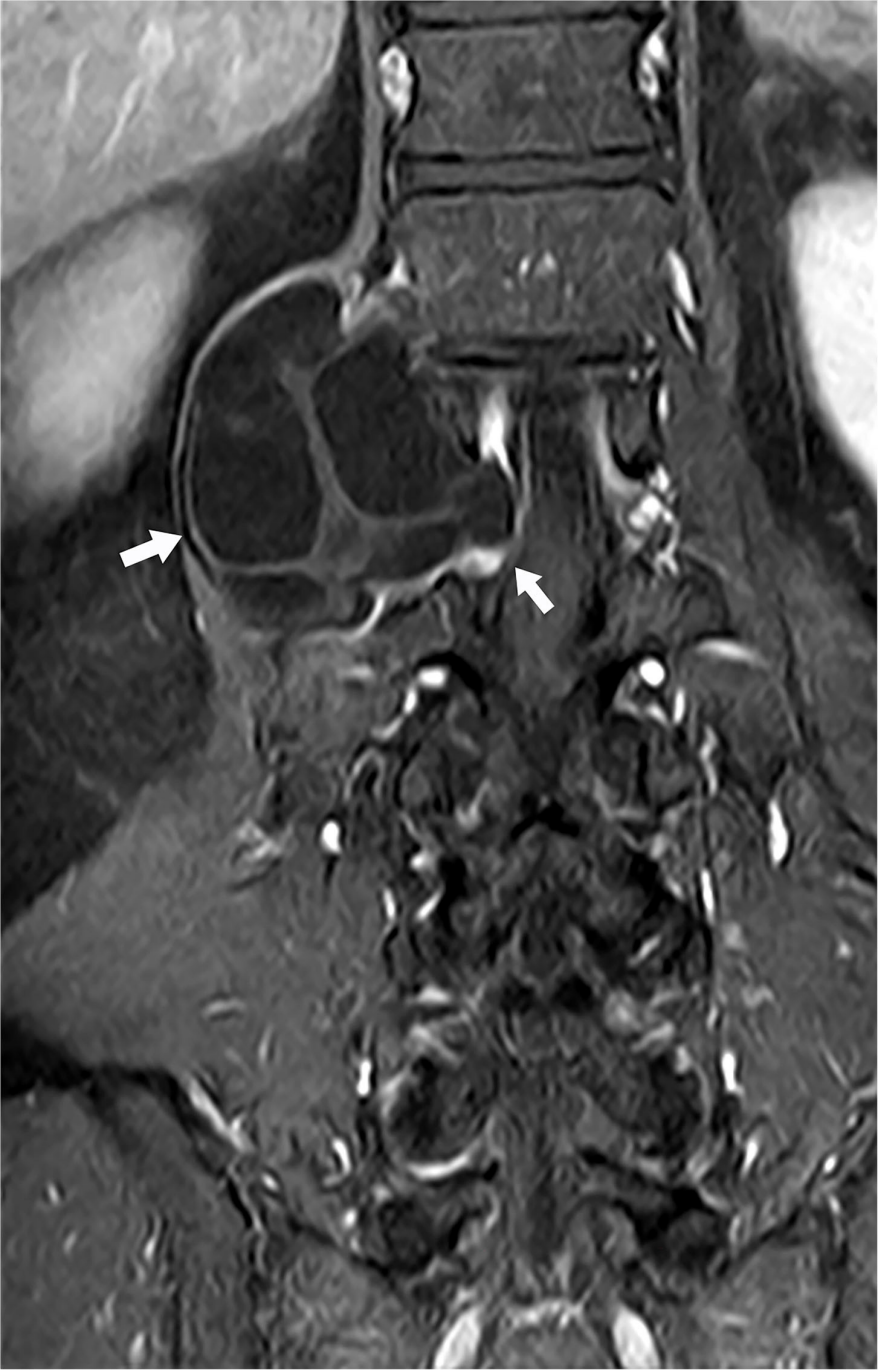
MRI T1WI (coronal image) showing corresponding intervertebral foramina around the cavity was enlarged. Left arrow: The part outside of the spinal canal. Right arrow: The part passing through the intervertebral foramen and inside of the spinal canal.
